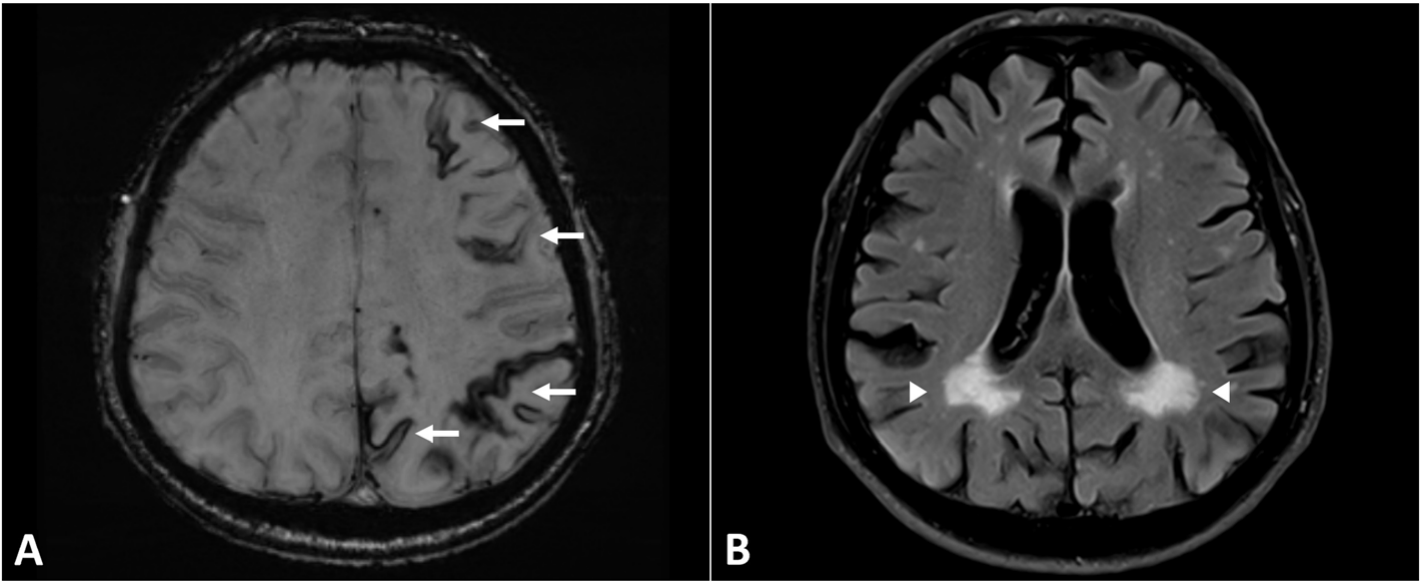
Follow-up neuroimaging findings at 1 year. Legend: Axial brain MRI with susceptibility-weighted imaging sequence showing that cortical superficial siderosis has been slightly restricted compared to the baseline MRI (Panel a, arrows). Axial brain MRI with fluid-attenuated inversion recovery sequence showing stabilization without further expansion of the symmetrical periventricular white matter hyperintensities compared to the baseline MRI (Panel b, arrowheads)
